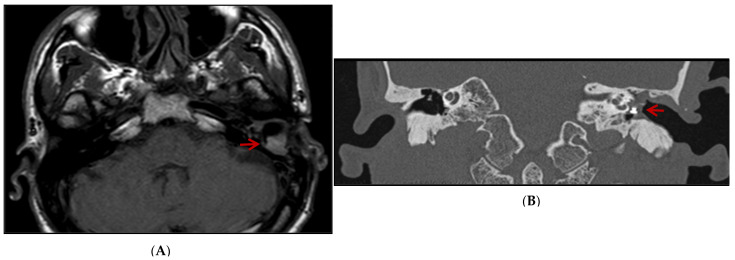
MRI axial (A) and CT scan coronal (B) images: scar lesion filling the left middle ear of the patient (highlighted by red arrow) compared to the right ear.
